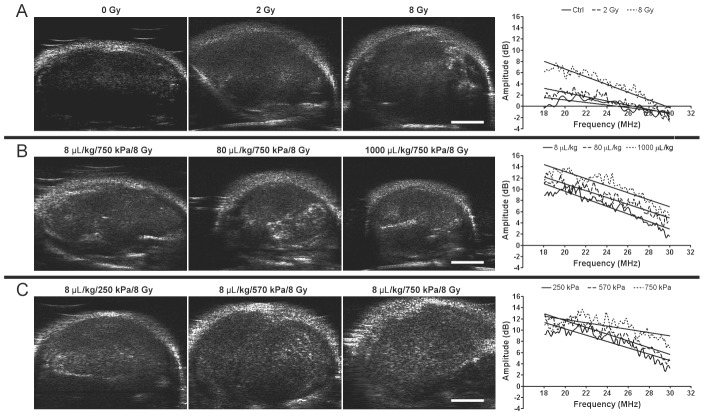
High-frequency ultrasound B-mode images of PC-3 xenografts. B-Mode (left) and on the right side of each row, respective representative normalized power spectra are shown. (A) Tumors treated with varying radiation doses (0–8 Gy) without ultrasound-microbubble treatment. (B) Tumors treated with varying microbubble concentrations (8–1000 µL/kg) combined with 750 kPa of ultrasound pulse and 8 Gy-radiation. (C) Tumors treated with varying ultrasound pressures (250–750 kPa) combined with 8 µL/kg of microbubbles and 8 Gy-radiation. The scale bar represents 2 mm.
